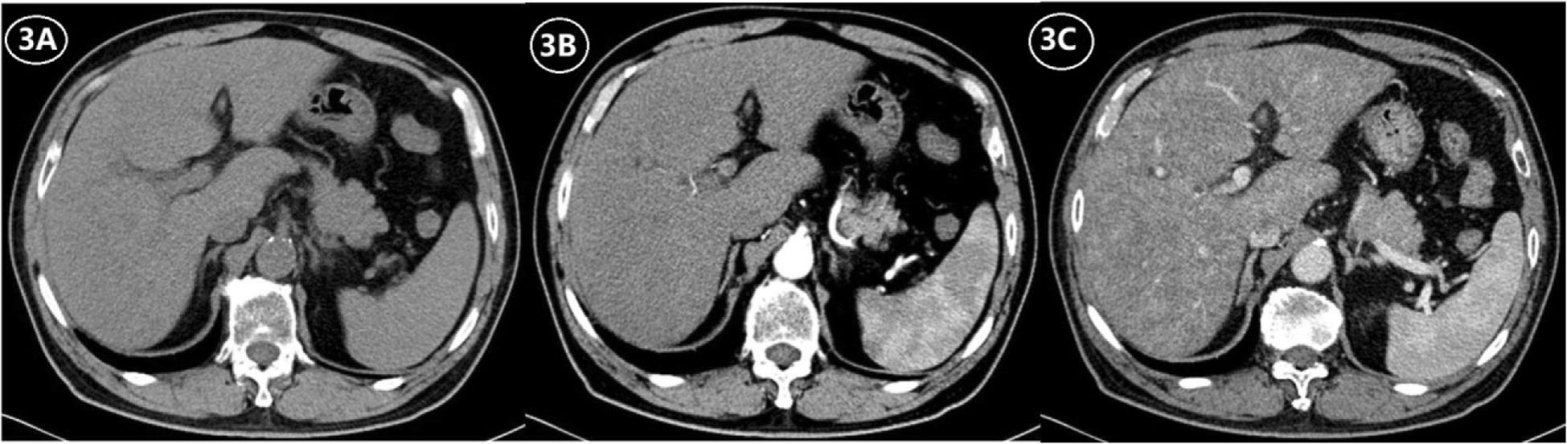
A 68-year-old man with SOS caused by Tu san qi. a Normal CT scan shows a non-uniform density of the liver parenchyma and mild hepatosplenomegaly. b Arterial phase contrast-enhanced CT scan shows a small and ill-defined hepatic artery and decrease of hepatic parenchyma enhancement. c Portal phase contrast-enhanced CT scan shows patchy low-density lesions with a non-uniform decrease of hepatic parenchyma enhancement (the CT value of the liver is lower than that of the spleen)
